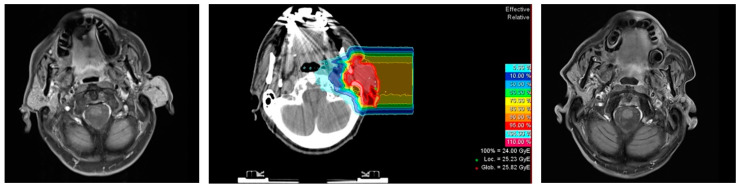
Example of patient with recurrent acinic cell carcinoma rcT2 cN0 cM0; left panel: contrast-enhanced T1-weighted MRI at diagnosis; middle panel: carbon-ion radiotherapy boost plan; right panel: contrast-enhanced T1-weighted MRI at follow-up with local control.
